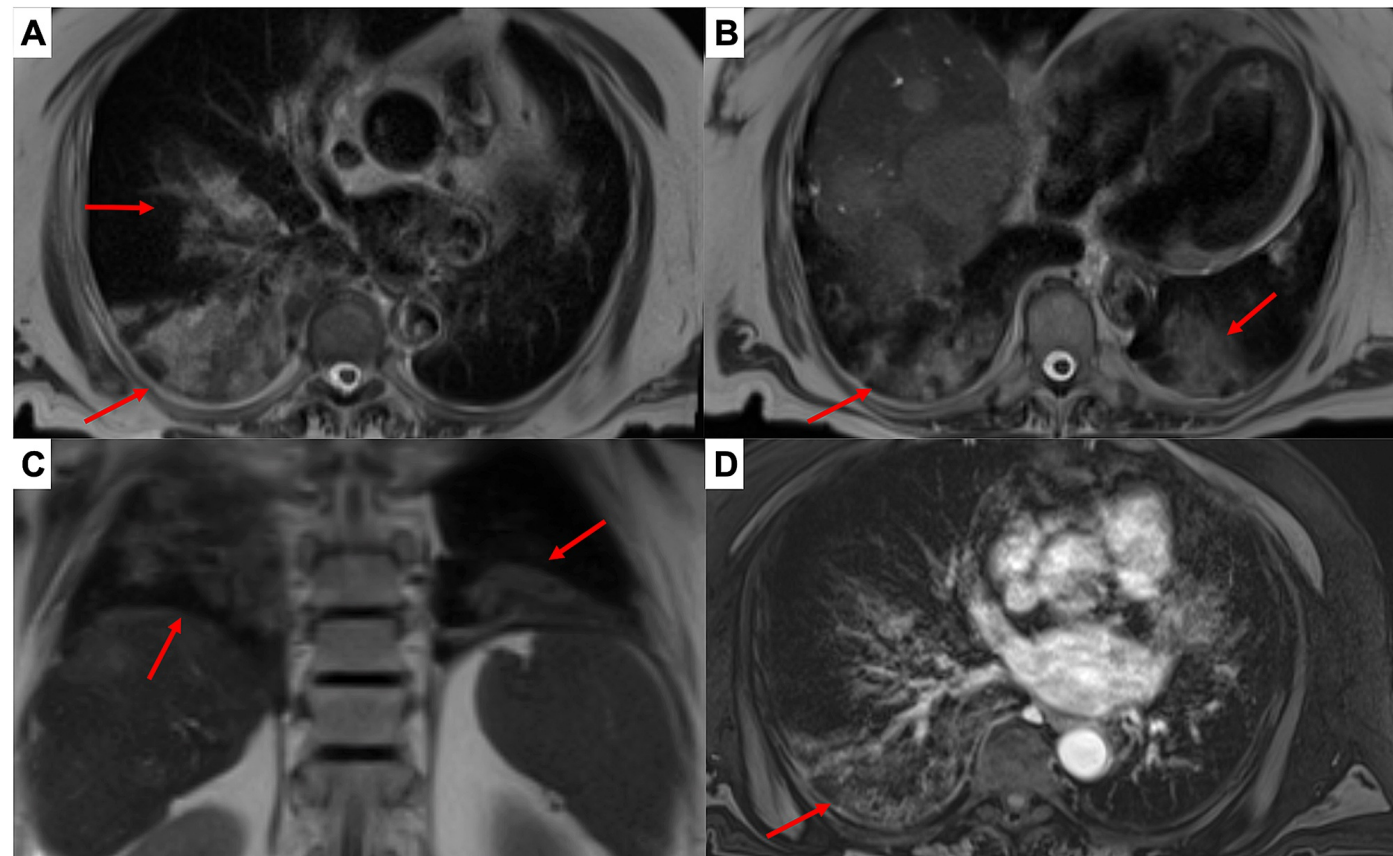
Magnetic resonance of the upper abdomen with a T2-weighted image on axial (A, B) and coronal (C) planes and T1-weighted 3D post-contrast images on axial plane (D). Areas of increased intensity with “ground glass” appearance (arrows) and superimposed inter-lobular and intra-lobular septal thickening were detected. The findings described are localized in both inferior lung lobes with a peripheral subpleural distribution.
